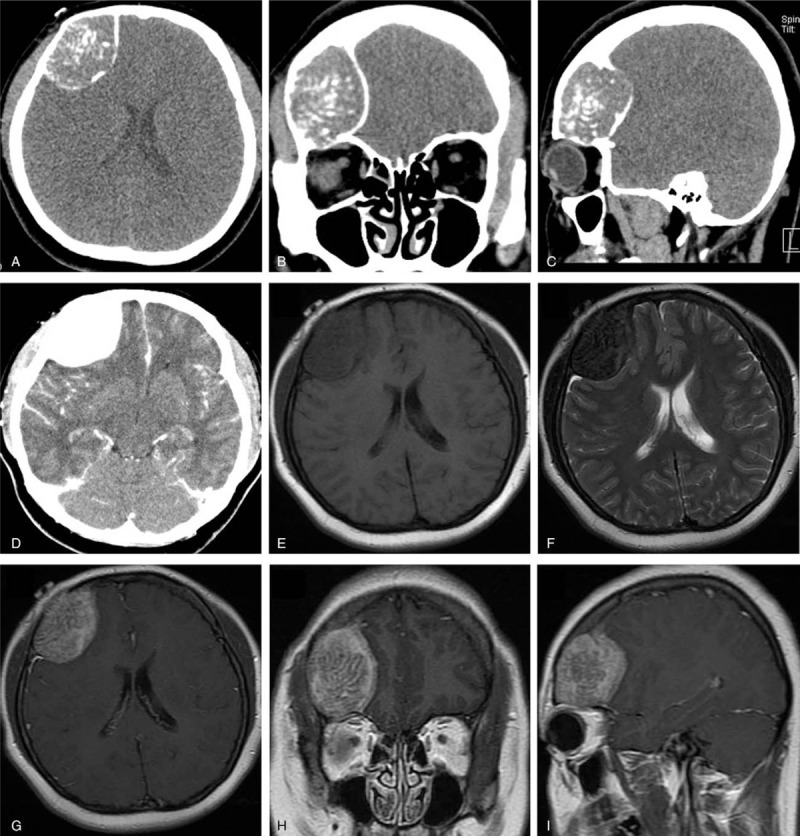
Preoperative computed tomography (CT) scan of the tumor in the frontal bone and orbital roof. (A–C) Preoperative CT of the head showed a 5 × 3.7 × 4 cm sized, well-demarcated osteolytic frontal lesion with mottled ossified density, which also infiltrated the orbital roof. (D) The result of enhanced CT, the lesion was shown to be with sufficient blood supply. (E–I) Enhanced magnetic resonance imaging magnetic resonance imaging revealed a solitary frontal mass, which was hypointense on T1- and T2-weighted images, and enhanced heterogeneously.
